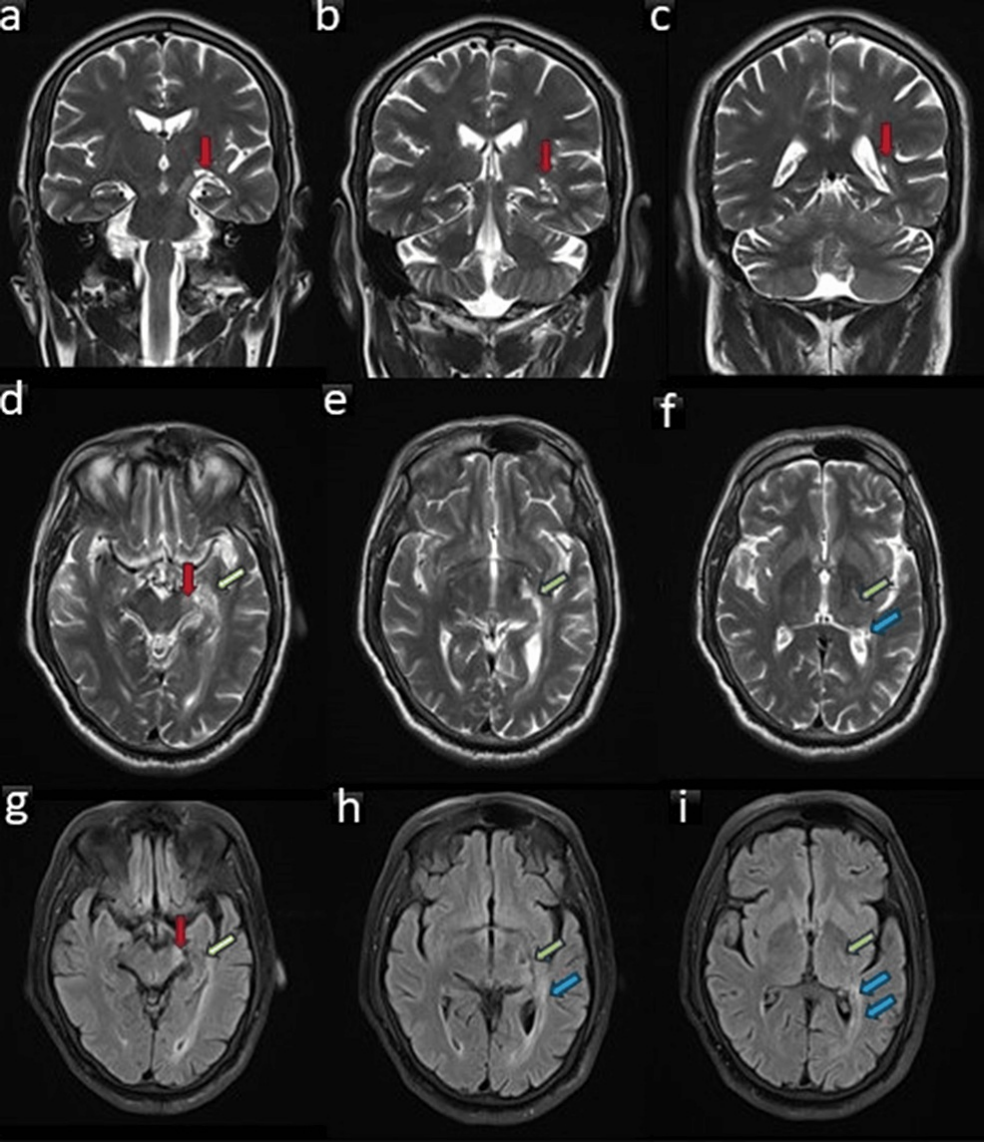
MRI images of the patient showing chronic infarct involving the left anterior choroidal artery territory infarct Coronal T2W brain MRI showing chronic infarct involving the left mesial temporal structures (arrows in a and b) and tail of caudate nucleus (arrow in c). Axial T2W brain MRI at the level of the midbrain (d), hypothalamus (e), and thalamus (f) showing chronic infarct involving the lateral aspect of the left cerebral peduncle (red arrow), mesial temporal structures (yellow arrow), lateral thalamus (green arrows), and optic radiation (blue arrows) on the left side.  FLAIR axial brain MRI images at the levels corresponding to d-f show chronic infarct in the areas as mentioned in images d-f. The infarct involving these areas is typical for anterior choroidal artery territory. FLAIR: fluid-attenuated inversion recovery
